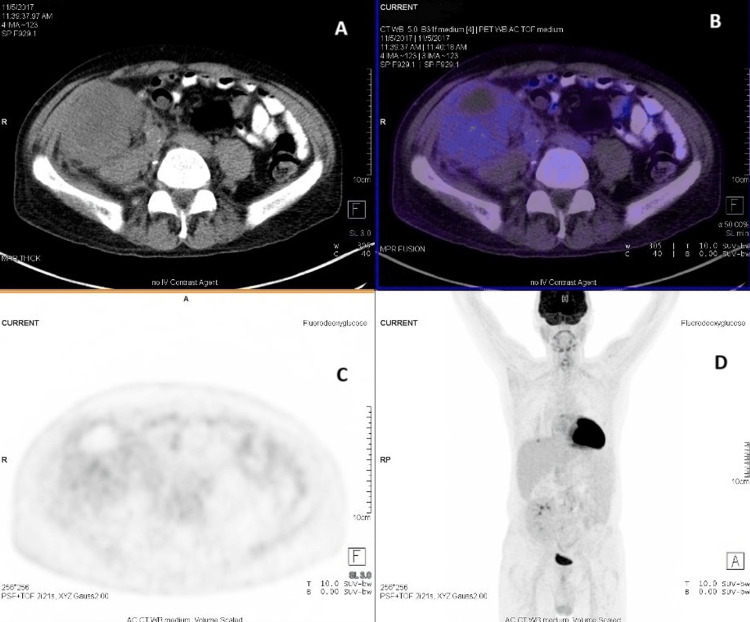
Case 1: PET-CT (A) The transplanted kidney is located in the right iliac fossa, showing decreased swelling (AP diameter reduced from 9 cm to 7 cm). (B) Evidence of FDG excretion indicates renal function. (C) Resolution of perinephric pathological soft tissue and enlarged paraaortic/right parailiac lymph nodes is observed. (D) No macroscopic signs of malignancy are suspected. FDG, fluorodeoxyglucose
